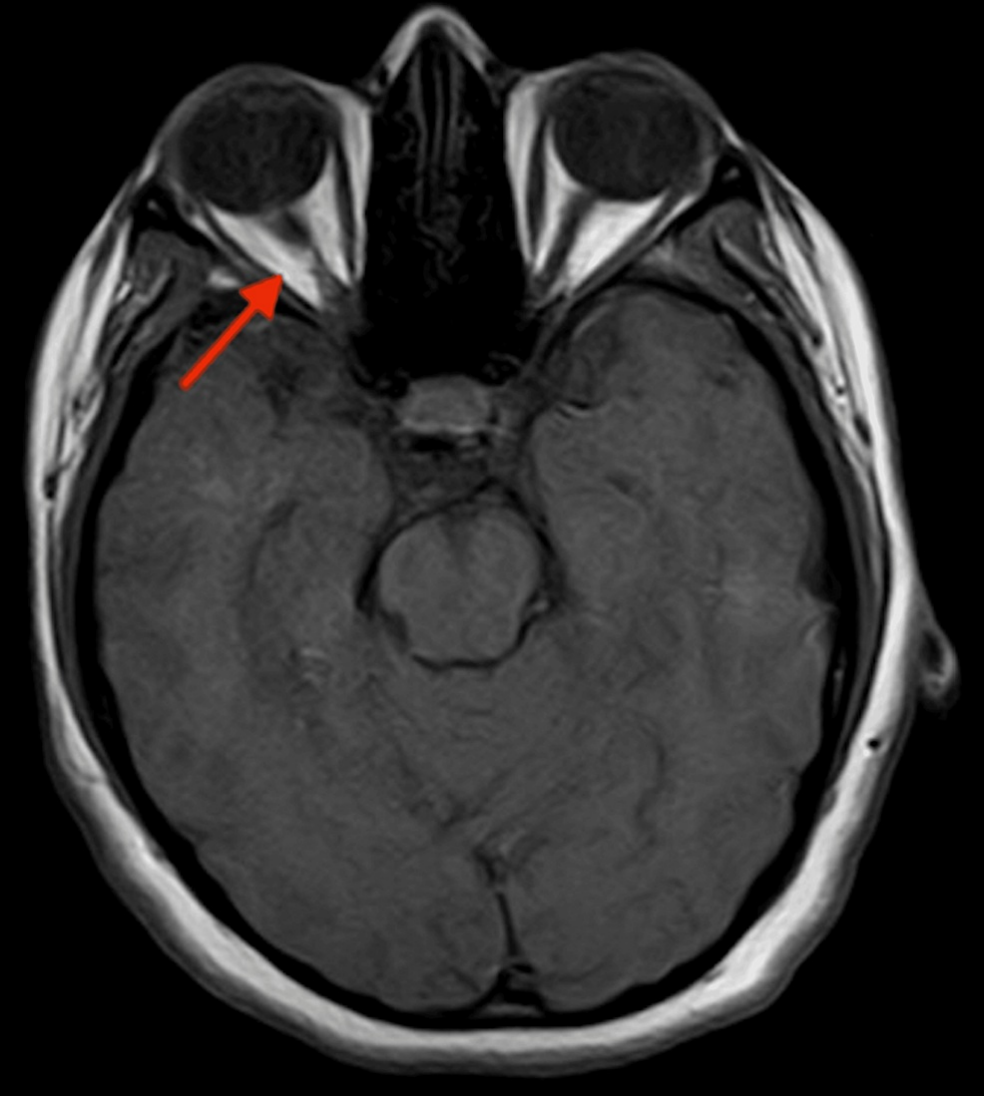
T1-weighted axial MRI with contrast of the skull and orbits showing enhancement of the right optic nerve. The image was negative for any other intracranial pathologies including white matter hyperintensities.
